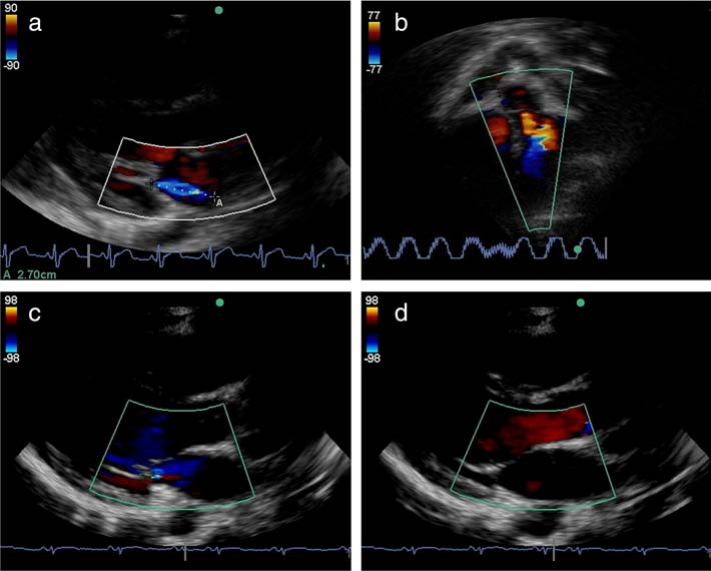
Examples of cardiac ultrasound performed by nurses. a. Parasternal long axis view demonstrating mitral regurgitation. This image was categorised adequate quality. b. Apical 5 chamber view demonstrating aortic regurgitation. The regurgitation was not identified by the nurse. This image was categorised poor quality. c and d. Parasternal long axis view demonstrating closing volume. Color is seen across the mitral valve in single frame at the time of valve closure, but not in mid-systole
